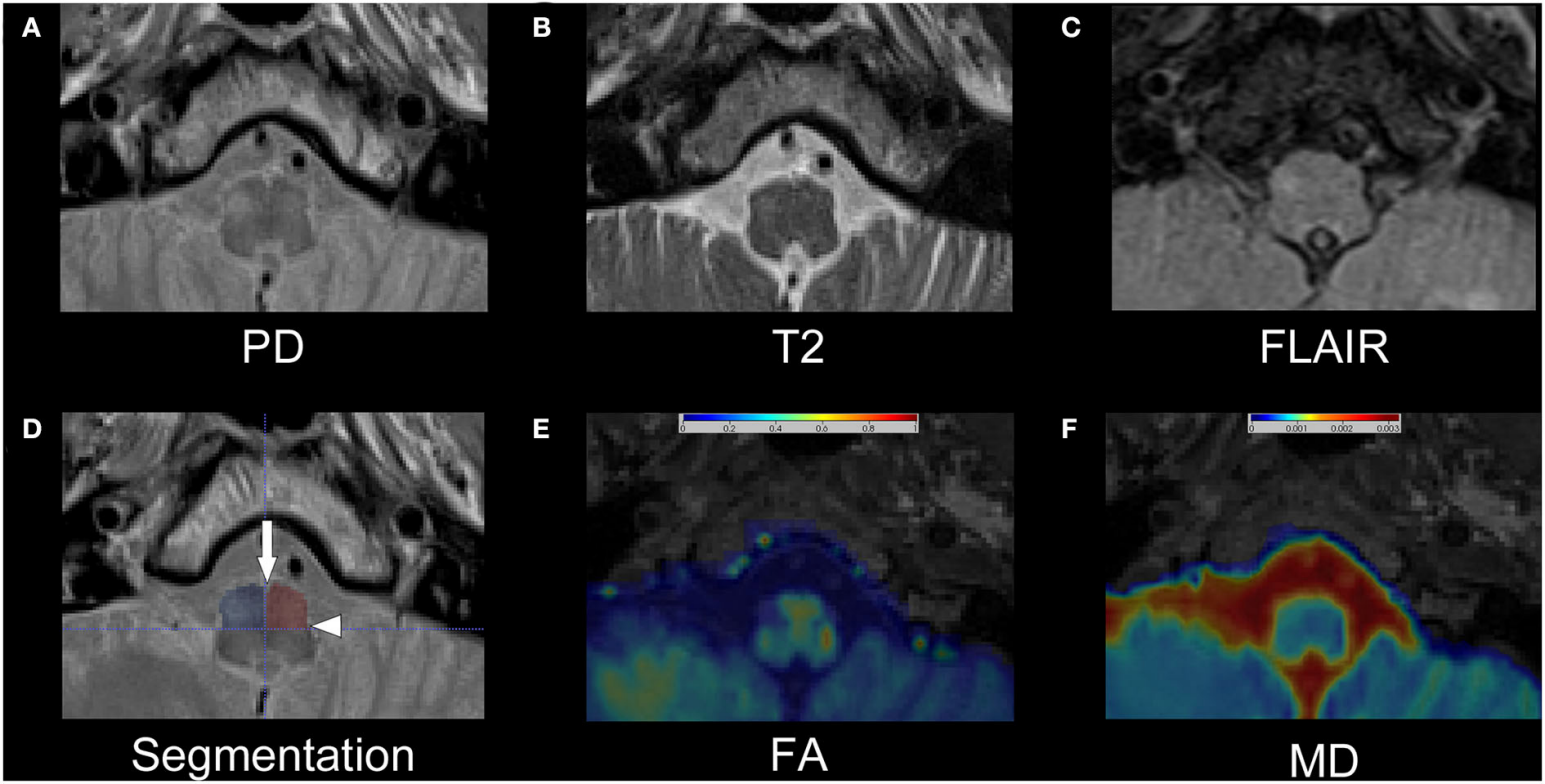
Exemplary dataset of patient number 13. The upper row shows the cropped PD-w (A), T2-w (B), and FLAIR (C) images that were used for the rater-based analysis. The segmentation of the anterior quadrants of the medulla oblongata is shown as semitransparent overlay on the PD-w image in (D) with an additional indication of the anterior median fissure (arrow) and the posterolateral sulcus (arrowhead). While the left ION is hardly visible, especially in the T2-w image and FLAIR, the right ION is hyperintense and possibly enlarged, indicating HOD. Images (E) and (F) show the corresponding FA and MD maps as interpolated and color-coded, semitransparent overlay on a T1-w image. In comparison with the left ION, a decrease in FA and an increase in MD are recognizable in the right ION. PD, proton-density; w, weighted; FLAIR, fluid-attenuated inversion recovery; ION, inferior olivary nucleus; HOD, hypertrophic olivary degeneration; FA, fractional anisotropy; MD, mean diffusivity.
